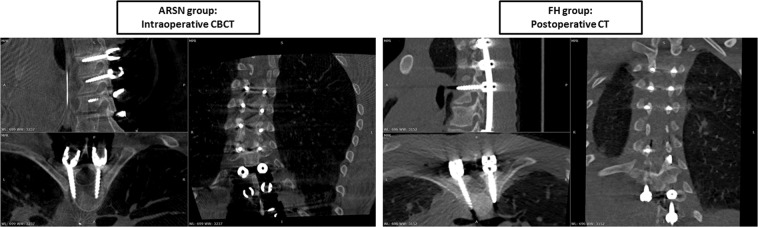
Examples of multi-planar images displayed according to each screw axis for screw position assessment. On the left, rating performed on an intraoperative cone beam CT of a patient treated with augmented reality surgical navigation (ARSN). On the right, rating performed on a postoperative CT of a patient treated with the free-hand (FH) technique.
